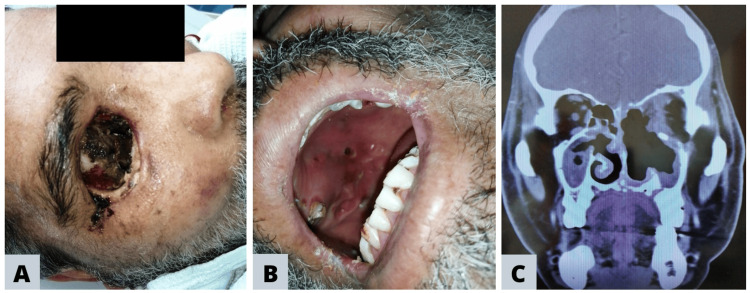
Clinical and Radiological Manifestations of Rhino-Orbital-Cerebral Mucormycosis Panels A and B depict the case of a 51-year-old male patient with stage 3 rhino-orbital-cerebral mucormycosis and uncontrolled diabetes (case number 16, supplemental material). In Panel A, the orbital basin displays inflammatory and necrotic tissue prior to surgical cleaning. Panel B highlights multiple necrotic ulcer lesions on the palate and teeth in a state of poor preservation and hygiene. Panel C offers a coronal view of computed tomography from a 58-year-old male patient with stage 4 rhino-orbital-cerebral mucormycosis and uncontrolled diabetes (case number 8, supplemental material). The image reveals the left maxillary sinus with dense heterogeneous tissue, lysis of the medial and posterior walls, frontal erosive bone lesions, and erosion of the lamina propria.
